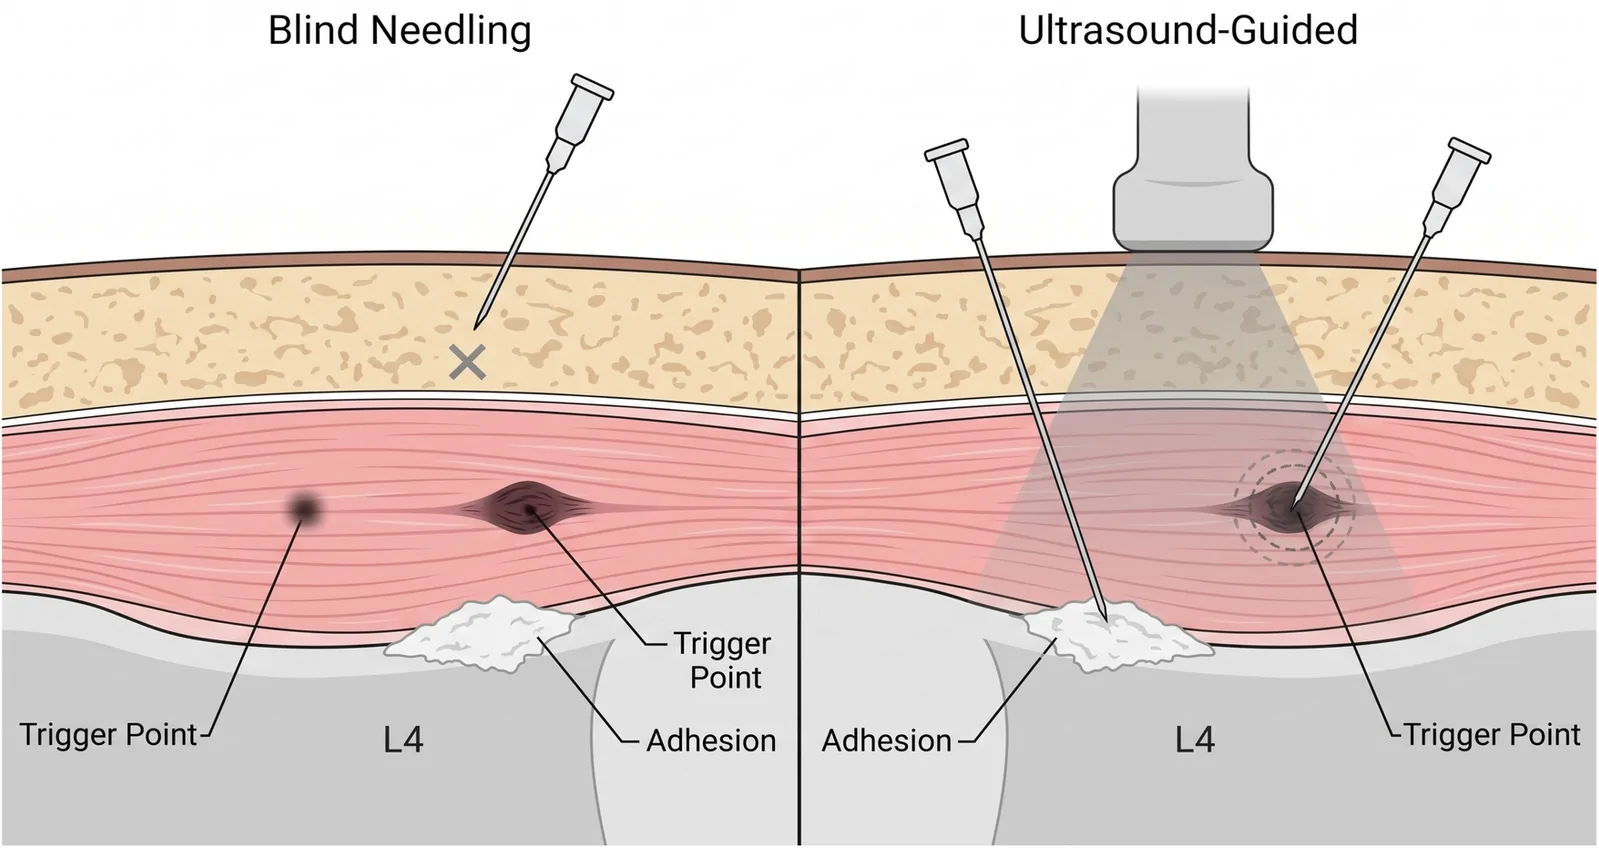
Blind vs. ultrasound-guided needling for deep pathological targets. Sagittal split-view comparison of the lumbar region. Left: blind needling terminates in subcutaneous fat, missing the periosteal adhesion and myofascial trigger point. Right: ultrasound guidance enables precise needle placement, with one tip releasing the adhesion and another eliciting a local twitch response (concentric ripples) within the trigger point. The gray wedge indicates the ultrasound scanning plane.
